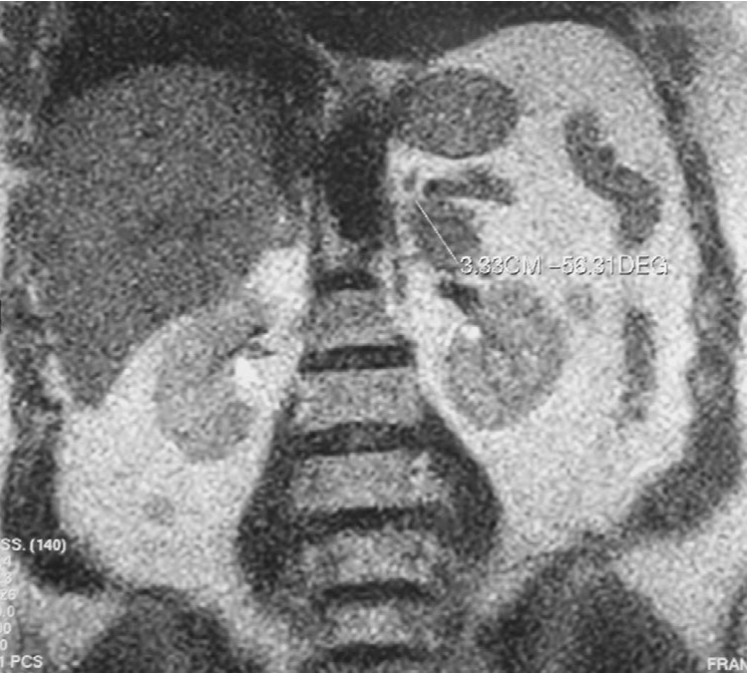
MRI T2-weighted imaging: central low-signal-intensity due to the extinguished cystic membrane and a more hypointensive rim of the cyst due to wall calcification.
